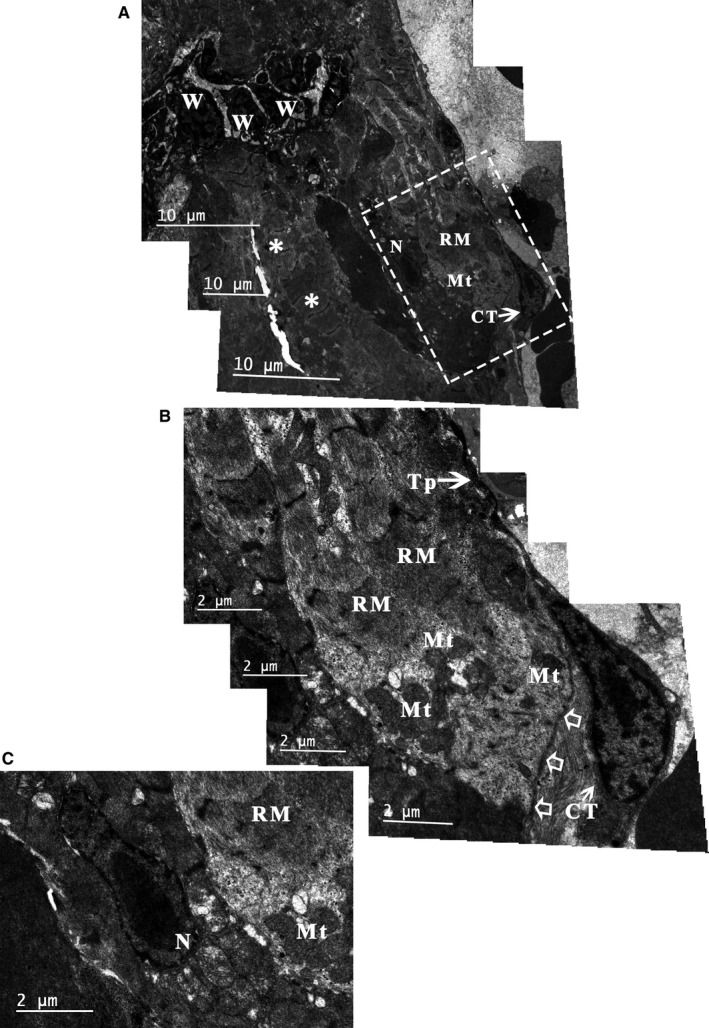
TEM analysis of the *Xenopus tropicalis* myocardium 8 d after injury. Eight days after injury, some of the injured muscle fibres regenerated via a novel muscle fibre characterized by an irregular muscle fibril arrangement and irregular sarcomere (RM) as well as regenerated sarcolemma (open arrow). An accumulation of mitochondria is seen in the regenerated muscle fibres (A, B), and a karyokinesis‐like nucleus was found in the border cardiomyocytes of regenerated myofibres (C). In addition, CTs with normal morphology are found on the outer surface of regenerated myofibres (A, B). B: Higher‐power view of the dotted line rectangle in A. Scale bar: Size as shown in the figures. Asterisk: Cardiomyocyte; CT: Cardiac telocyte; Mt: Mitochondria; N: Nucleus; RM: Regenerated myofibres; Tp: Telopode; W: Inflammatory cell
